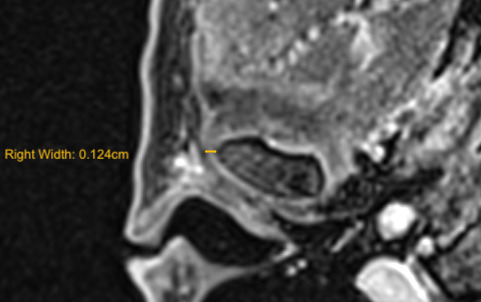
MRI of a right temporomandibular joint in the axial contrast enhanced Magnetic Resonance image (*T*_1_ weighted TSE).The LPAS width was measured from the reference point of the hypointense signal of the most lateral cortical of the condyle to the external limit of the periarticular tissue. LPAS, lateral periarticular space; TSE, turbo spin echo.
